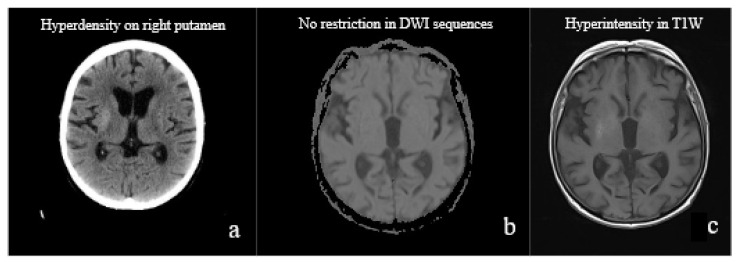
Imaging findings. (a) Brain CT (28/01/2022) with evidence of a hyperdense alteration on the right putamen. (b,c) MRI (10/05/2022) in DWI and T1W sequences with evidence of a persistent hyperintense lesion on the right putamen and no sign of restriction in diffusion sequences.
